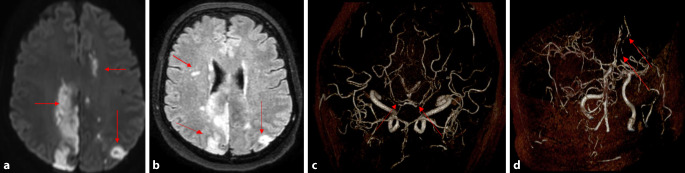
Magnetresonanztomographie (*MRT*) des Neurokraniums; a, b *DWI* („diffusion weighted imaging“) und FLAIR („fluid-attenuated inversion recovery“) zeigen mehrere multiple frische, allerdings bereits demarkierte Läsionen in mehreren Stromgebieten (*Pfeile*); c MRT(Magnetresonanztomographie)-Angiographie mit bilateralen Stenosen der A. cerebri posterior bds. (*Pfeile*); d MRT-Angiographie mit bilateralen Stenosen der A. cerebri anterior bds. (*Pfeile*)
